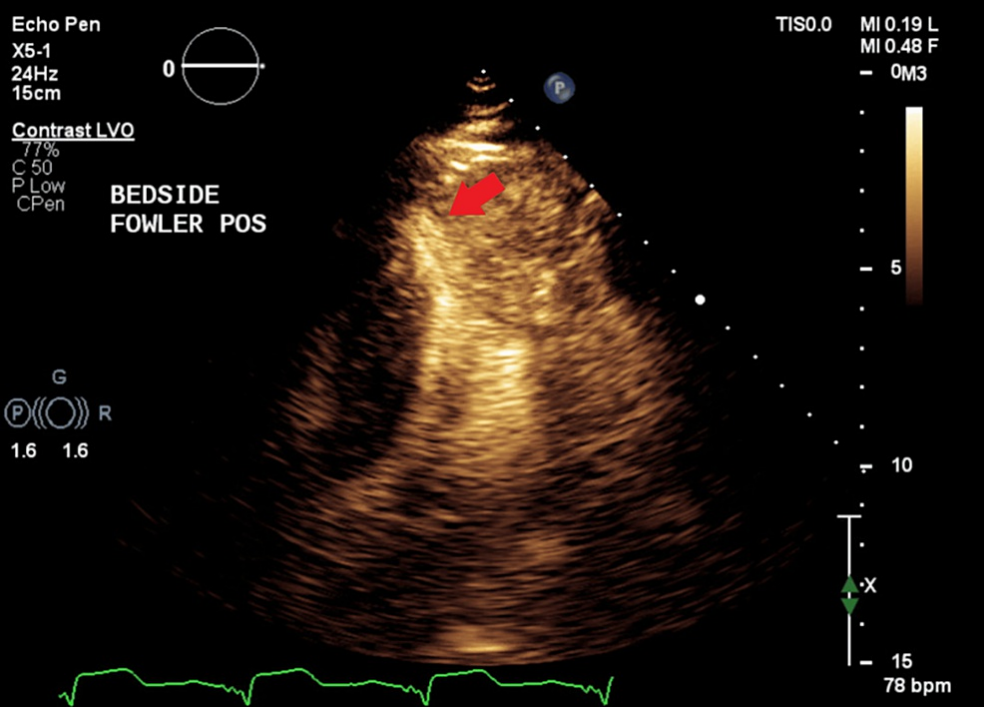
Transthoracic echocardiogram-apical view Apical thickening
